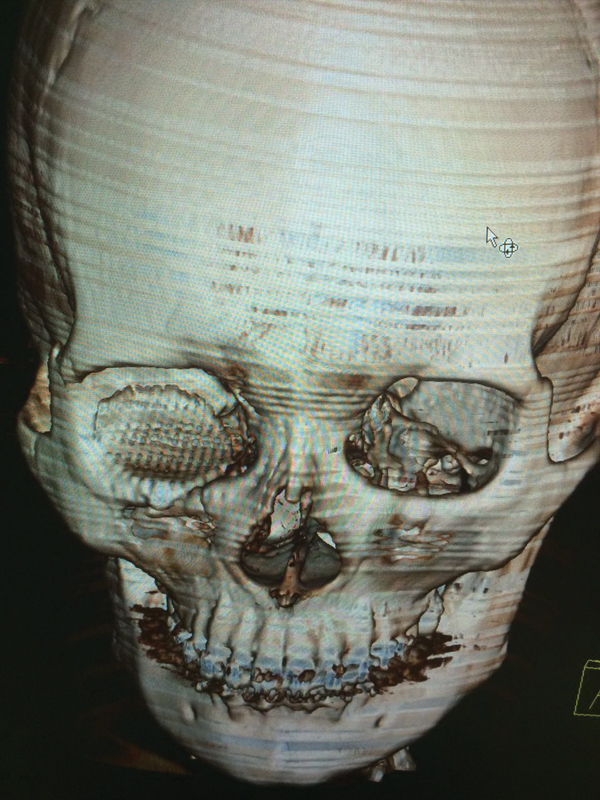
Three-dimensional computed tomographic (3D CT) scan 4 months
